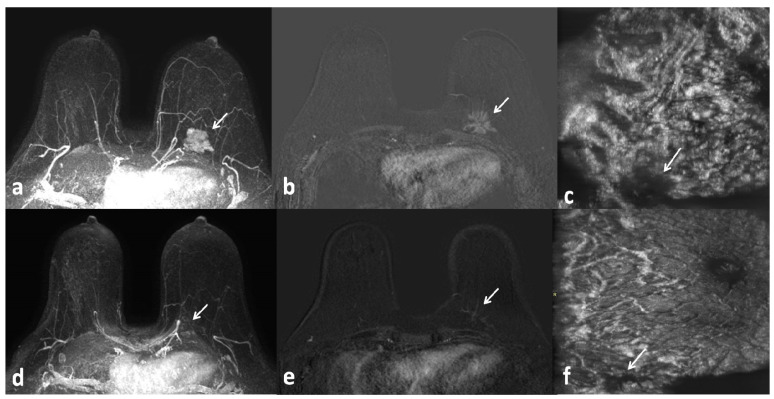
Axial MRI with maximum intensity projection (MIP) reconstructions (a,d), subtracted (post-contrast–pre-contrast) images (b,e), and ABUS coronal images (c,f) taken before (a–c) and after (d–f) NAC. Triple-negative cancer of the left breast with partial response to NAC (arrows).
